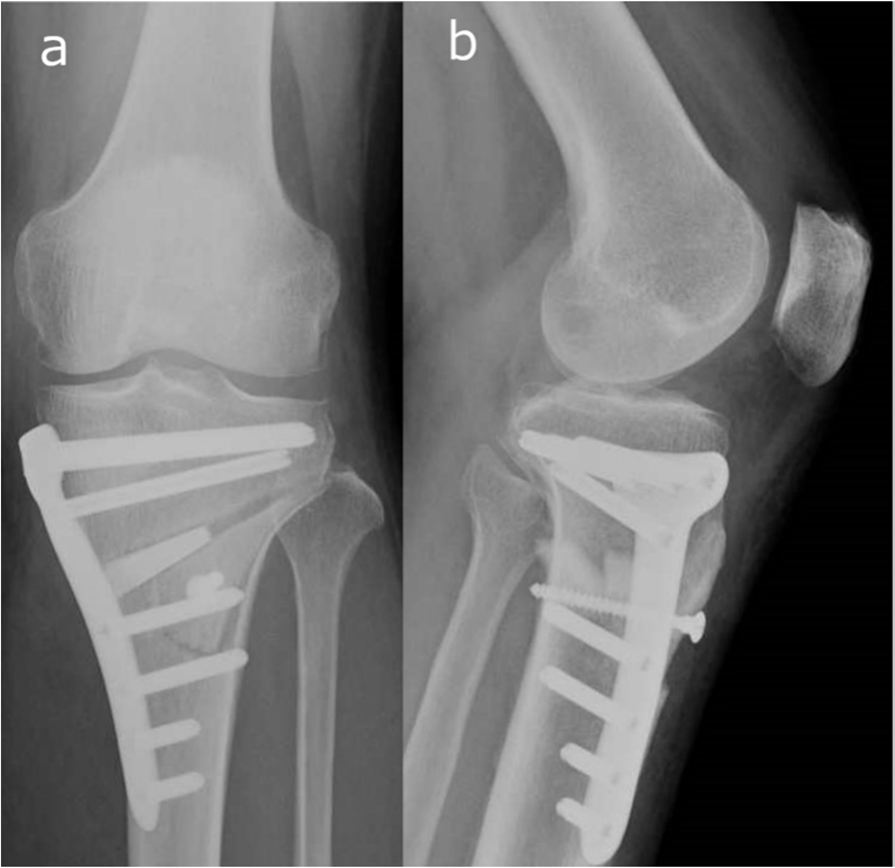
Radiographs after medial open-wedge DTO. A Anteroposterior radiograph. B Lateral radiograph
